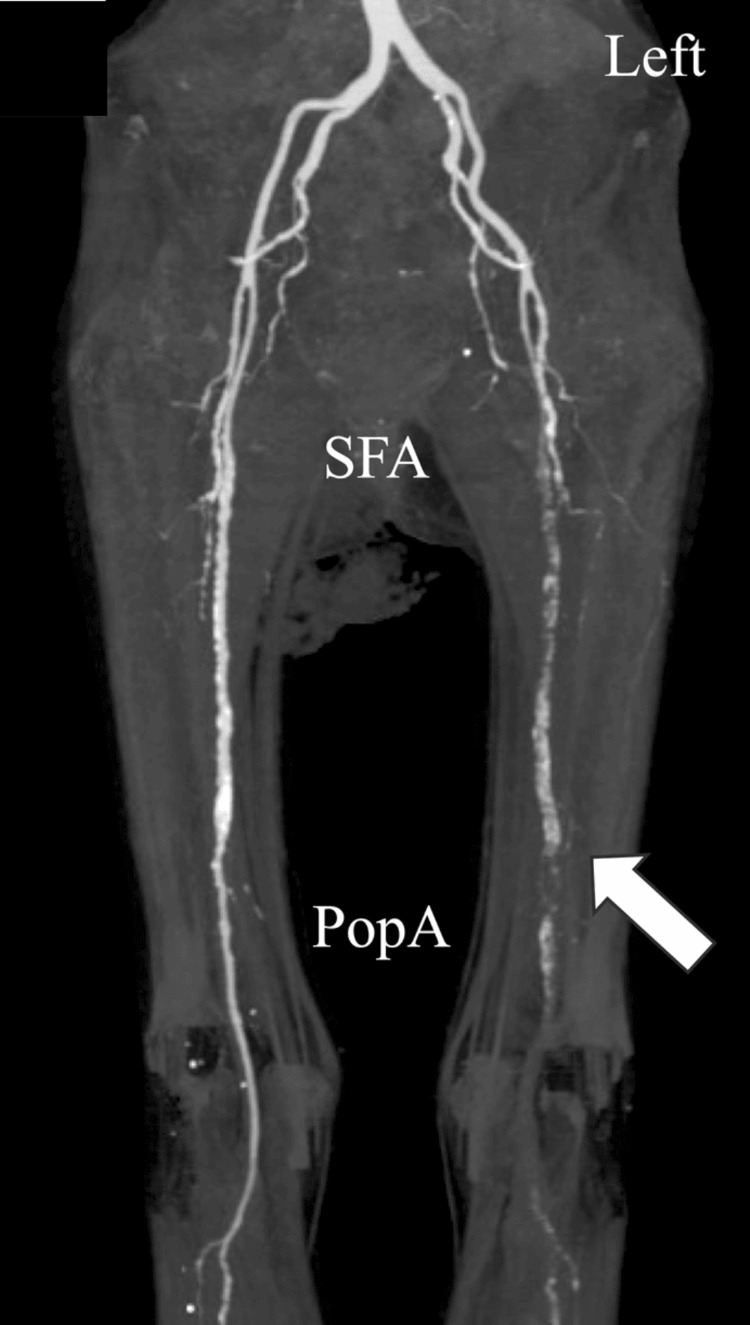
Contrast-enhanced CT performed on day 7 shows obstruction of the left popliteal artery (white arrow) PopA, popliteal artery; SFA, superficial femoral artery
